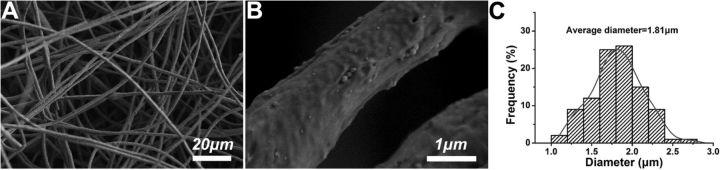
SEM images and fiber diameter distribution of dual-drug-loaded PLGA/FLU/RHB-loaded MSNs electrospun fibers after exposed to ultrasound irradiation
